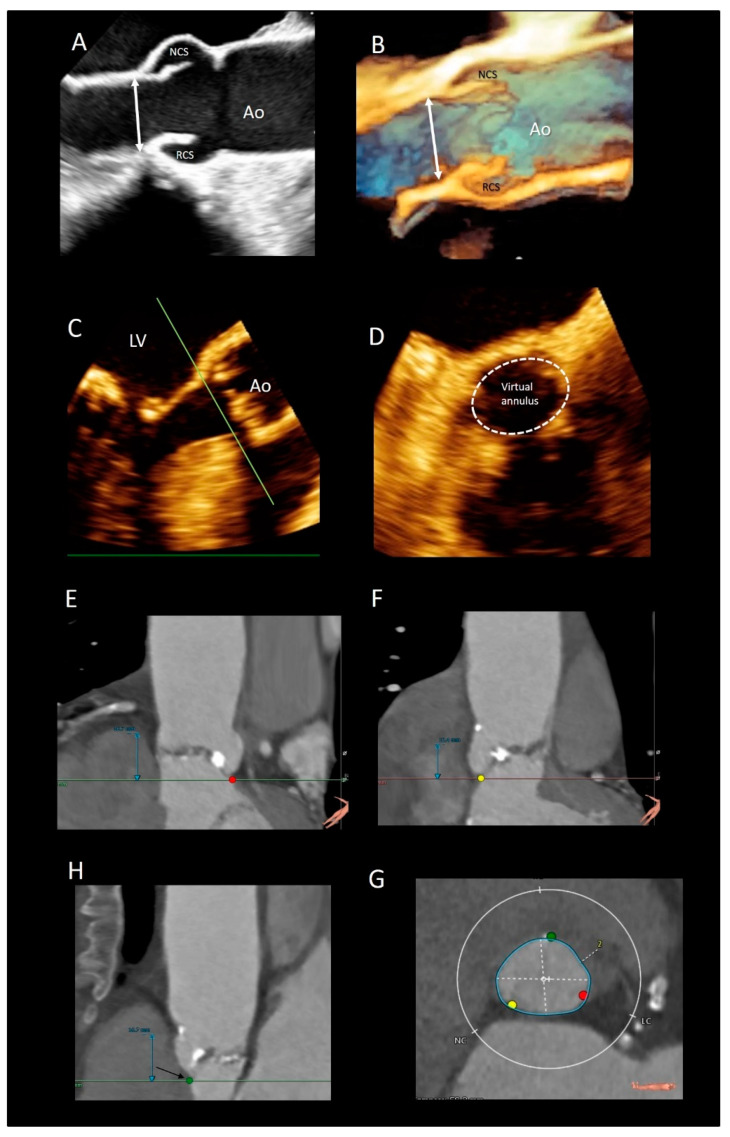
(A) The 2D and (B) 3D TEE long-axis view showing the echocardiographic annulus. (C,D) Multiplanar reconstruction using the 3D data set showing the virtual annulus. (E,F,H) CT scans showing the 3 nadirs (marked by the red, green, and yellow circles). (G) Dedicated software aligns the three nadirs in the same plane making possible measurements of diameters (white dotted lines) and circumference (blue line).
